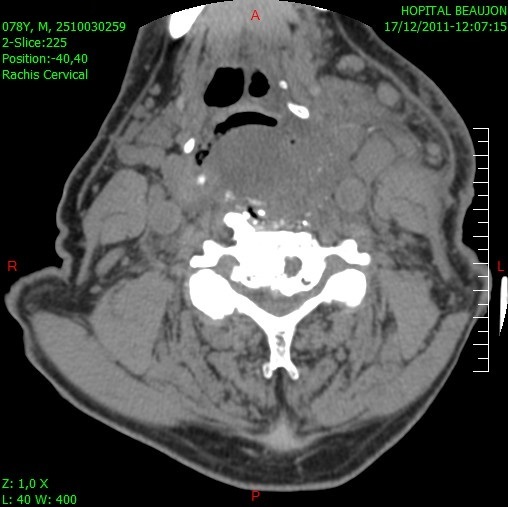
Postoperative sagittal cervical CT scan with bone windowing showing complete resection of anterior osteophytes
